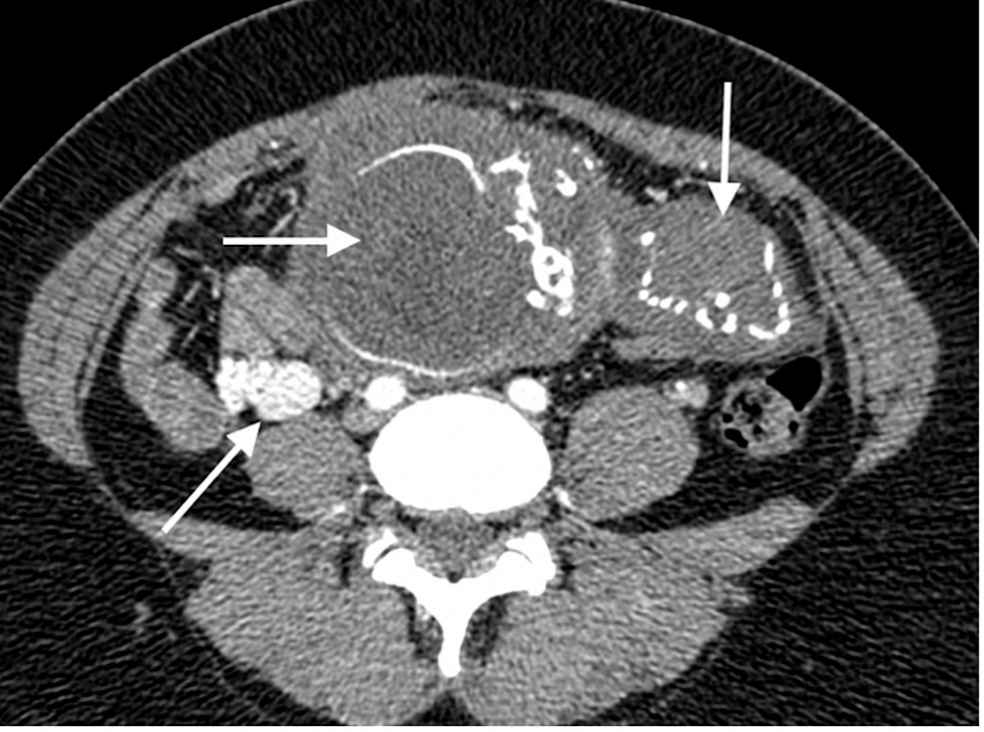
Axial, post-contrast CT, arterial phase demonstrating fetal head with hydrocephalus (horizontal arrow), fetal thoracic cavity located within the left side of the maternal abdomen (vertical arrow), and early filling of the engorged right ovarian vein (oblique arrow).
